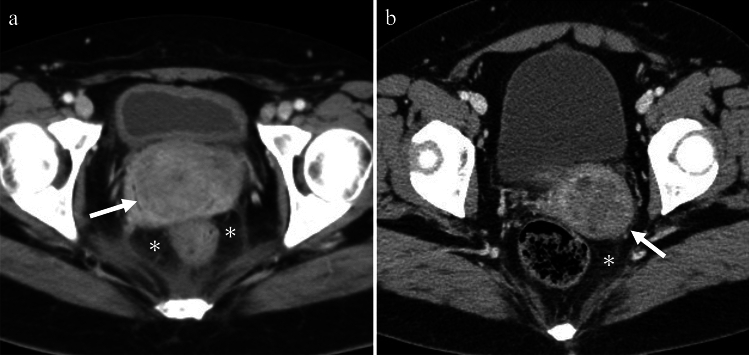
a Woman in her 40 s with lower abdominal pain who tested positive for Chlamydia Enlarged edematous uterine cervix (arrow) with fat stranding (asterisks) on contrast-enhanced CT indicates cervicitis. b Woman in her 40 s with abdominal pain who tested positive for Chlamydia Multiple cysts with heterogeneous attenuation (arrow), indicating cystic cervicitis are observed. A slight fat haziness (asterisk) is also observed
